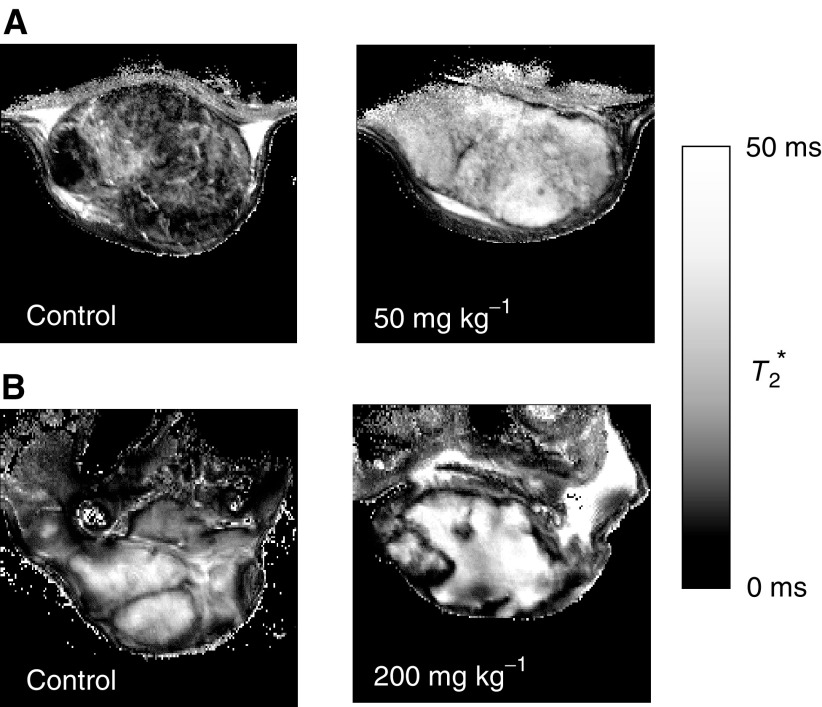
Calculated *T*_2_^*^ maps of (A) control and treated GH3 prolactinomas and (B) control and treated RIF-1 fibrosarcomas acquired as part of the MGRE MRI efficacy study. *T*_2_^*^ maps, rather than *R*_2_^*^ (=1/*T*_2_^*^) maps, are shown for clarity. Treatment with ZD6126 resulted in an increase in the signal intensity of the *T*_2_^*^ maps of both tumours, consistent with a decrease in tissue [deoxyhaemoglobin].
